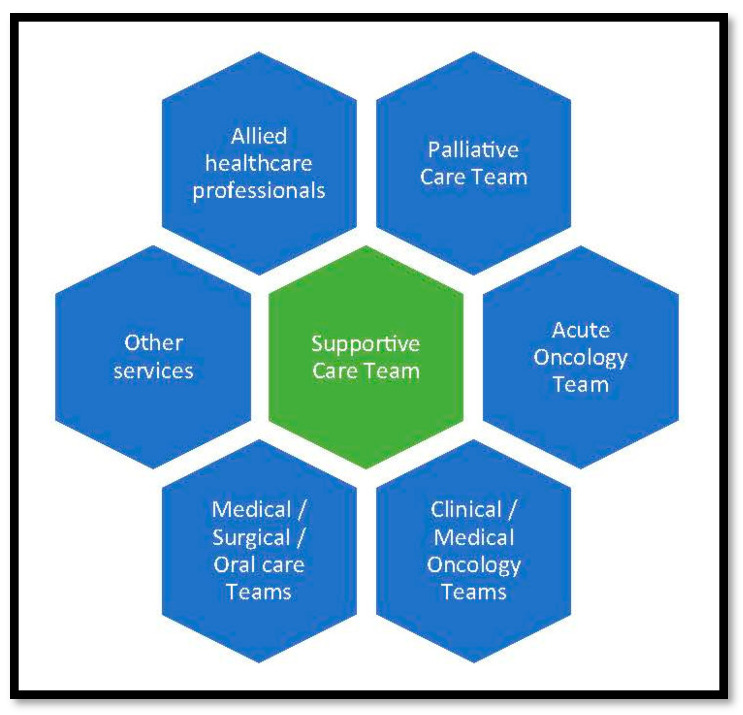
The “extended” supportive care team [5]. “Other services” include (but are not confined to) psychology, social work, and pastoral care services. “Allied healthcare professionals” include (but are not confined to) physiotherapists, occupational therapists, dieticians, and radiographers.
